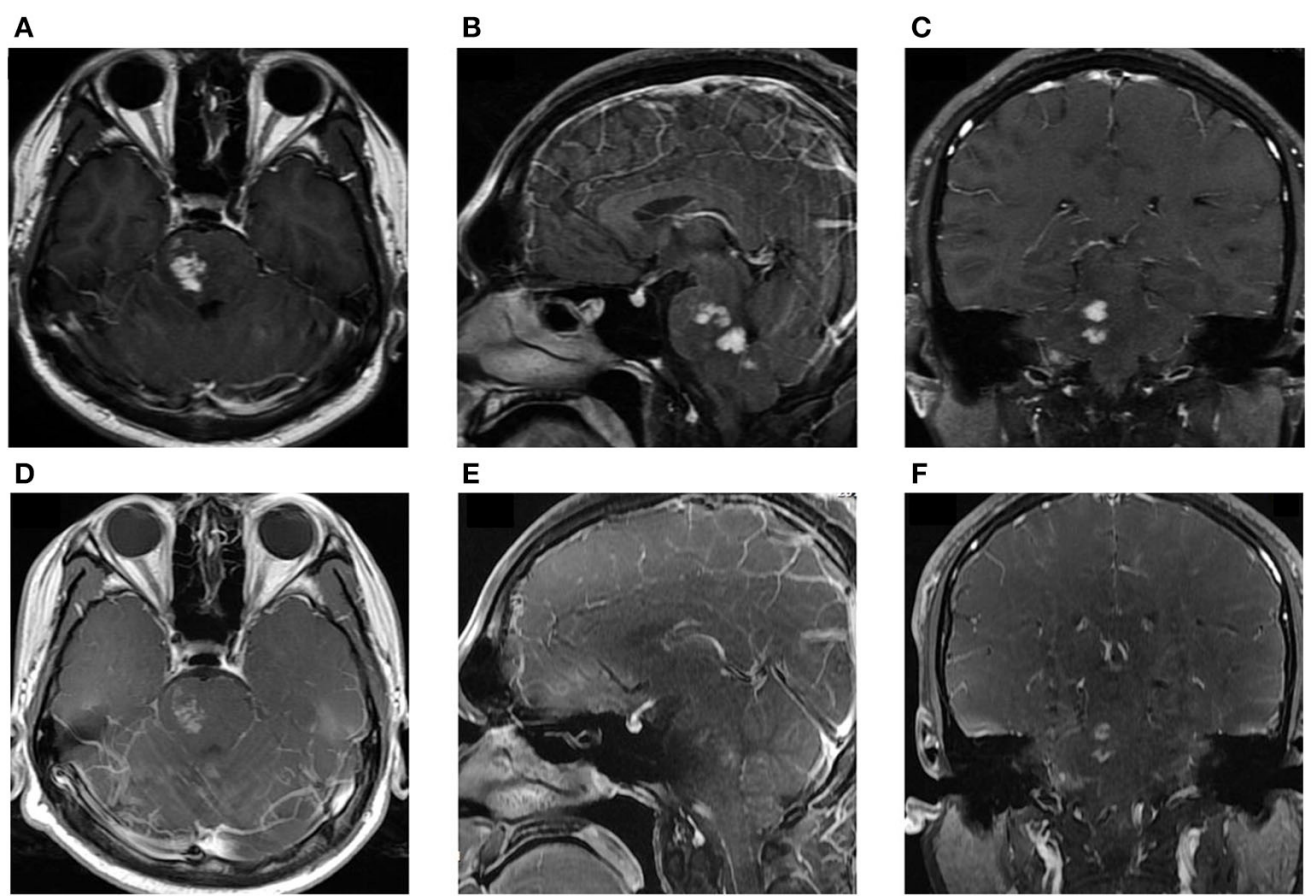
(A–C) MRI at admission: Axial, sagittal, and coronal T1-weighted, enhanced images showing significant, patchy, marked-enhancement lesions in the right brainstem after intravenous gadolinium administration. (D–F) Post-treatment MRI at the 4-month follow-up: T1-weighted enhanced images showing regressive lesions in the brainstem.
